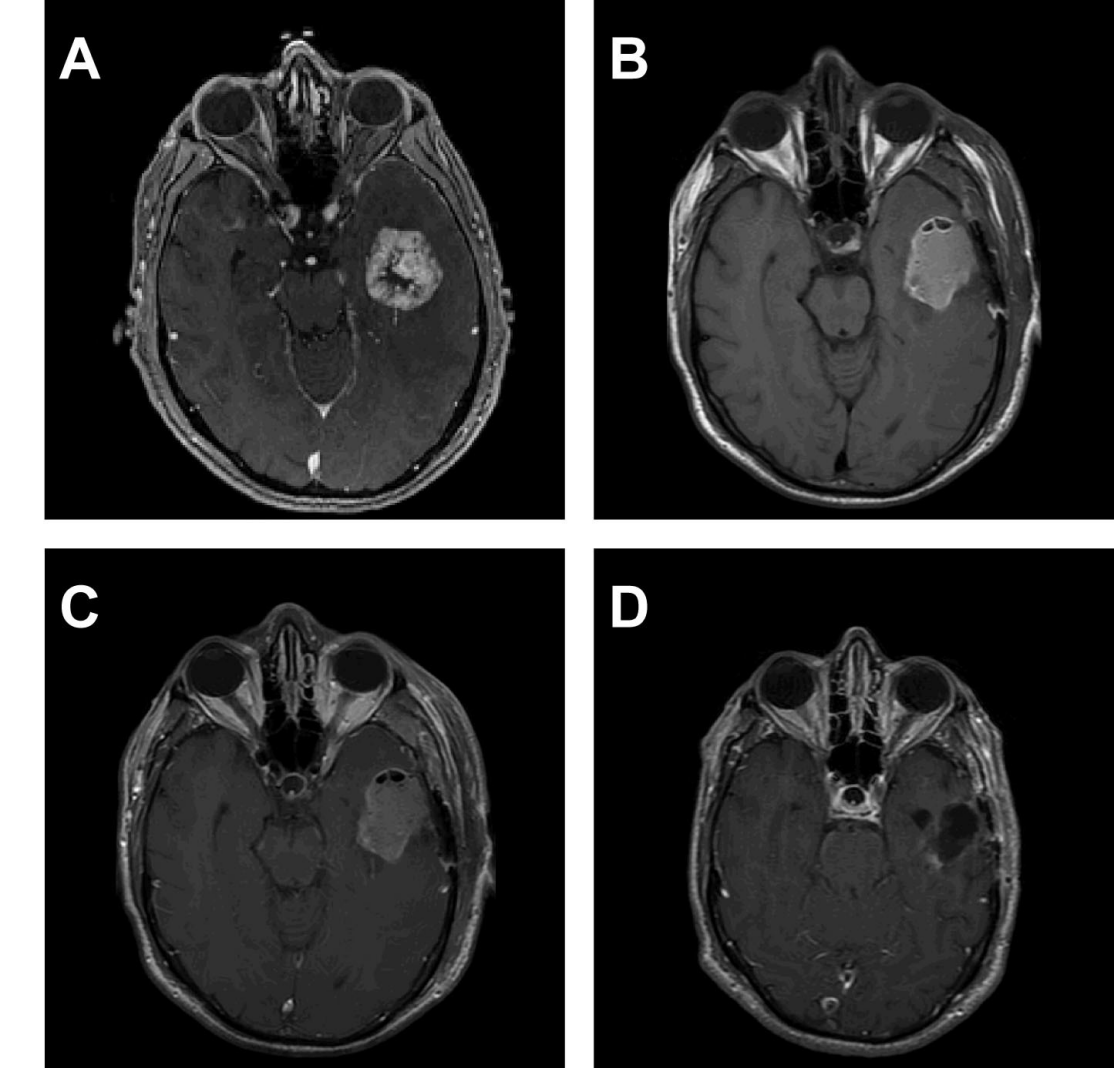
Case Example 2 This 58-year-old male underwent gross total resection of a left temporal renal cell carcinoma metastasis with planned Cyberknife radiosurgery. (A) Preoperative contrast enhanced T1 weighted MRI. Initial postoperative T1 weighted imaging (B) showed a largely blood filled resection cavity with a volume of 18.1 cm^3^. There was no residual enhancement seen on a contrast enhanced T1 weighted image. A pre-SRS planning MRI was performed 59 days later and revealed a resection cavity of 5.9 cm^3^ (67% volume reduction) with no evidence of tumor progression on contrast enhanced T1 weighted imaging (D).
